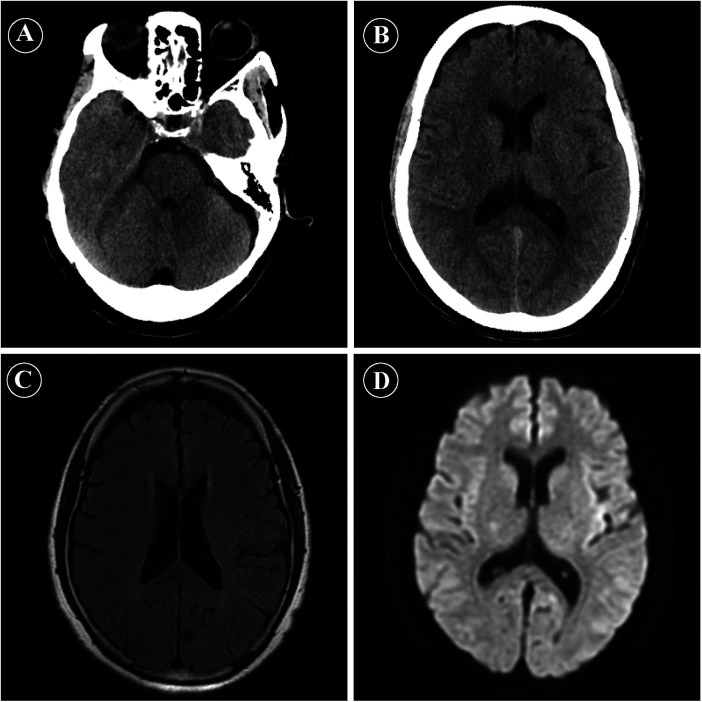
Emergency neuroimaging findings after coronary angiography. (A,B) Non-contrast cranial computed tomography (CT) images obtained shortly after symptom onset, showing no evidence of intracranial hemorrhage or acute ischemic lesions. (C) Axial fluid-attenuated inversion recovery (FLAIR) magnetic resonance imaging (MRI) showing no abnormal signal intensity in the bilateral occipital lobes or other brain regions. (D) Diffusion-weighted imaging (DWI) revealing no diffusion restriction, effectively excluding acute cerebral infarction.
